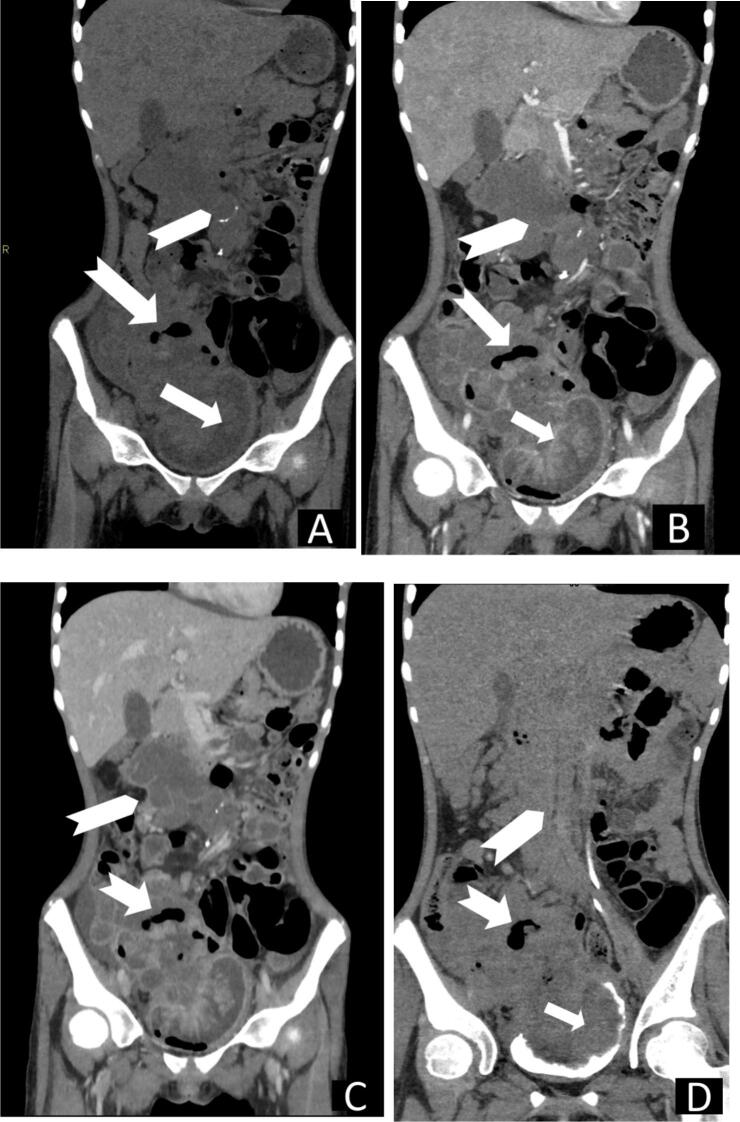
Computed tomography (CT), coronal reconstructed images (A) pre-contrast, (B) arterial phase, (C) portal venous phase and (D) delayed images revealed a right lower abdominal mass (arrows) extending from the urinary bladder through the right superior and lateral wall, breaching the bladder wall and almost filling the lumen. There is associated distal ileal loop inflammation, fistulous formation between bladder and bowel loops and air-filled cavity within the mass (notched white arrows), and intraabdominal adenopathy and calcification (white chevron).
